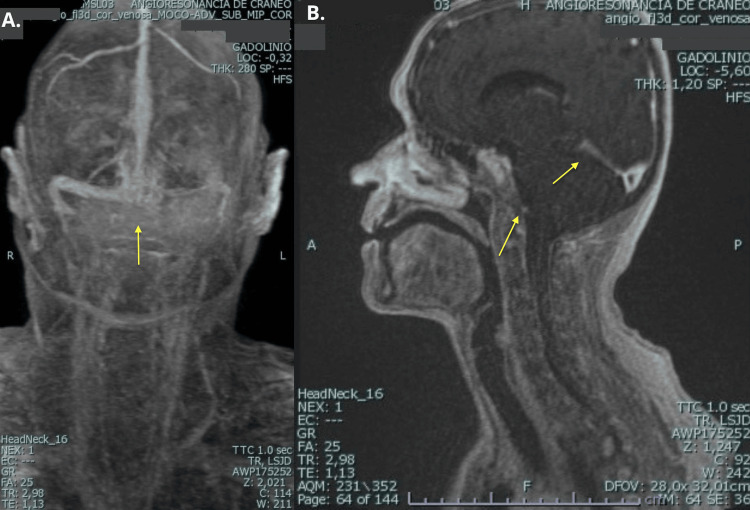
MRI and MRV findings MRA of the head, venous phase (MRV). (A) Coronal MIP reconstruction demonstrating the absence of normal flow and a distinct filling defect within the straight sinus, extending from the deep venous system. (B) Contrast-enhanced (gadolinium-enhanced) T1-weighted sagittal view showing signal alteration and thickening secondary to thrombosis involving the straight sinus, vein of Galen, and internal cerebral veins. MRA: magnetic resonance angiography, MRI: magnetic resonance imaging, MIP: maximum intensity projection
